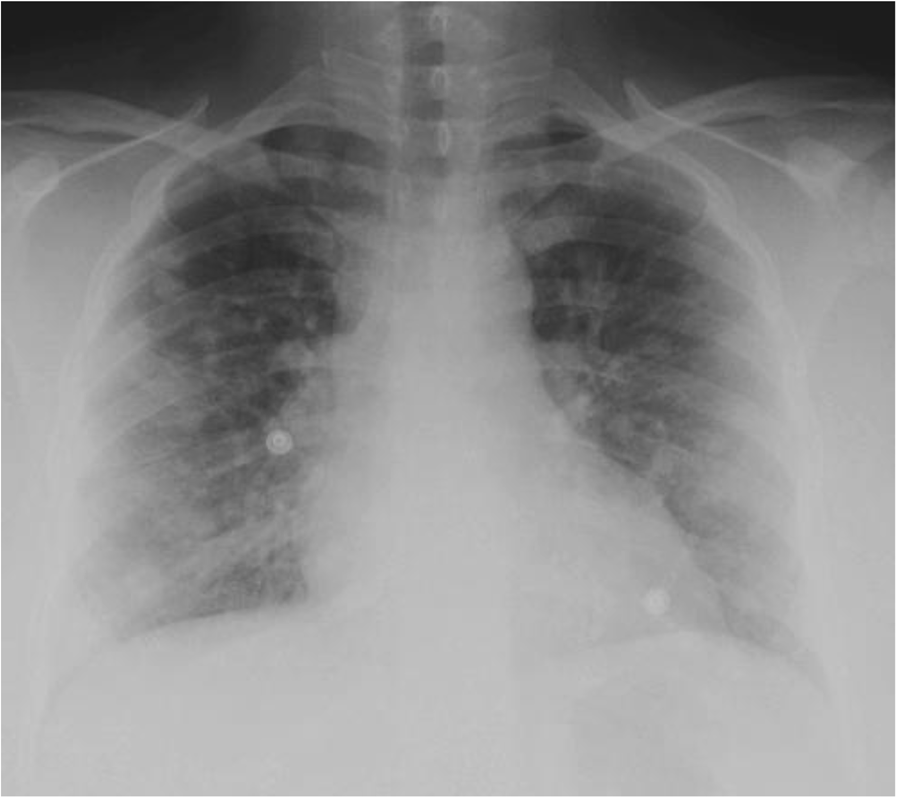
Chest X-ray of Case 1: A 32 year- old female presented with a 2-month history of fever, dry cough, shortness of breath on exertion and weight loss. She was treated with a number of antibiotics with no significant improvement. Chest radiograph showed extensive bilateral nodular infiltrates.
